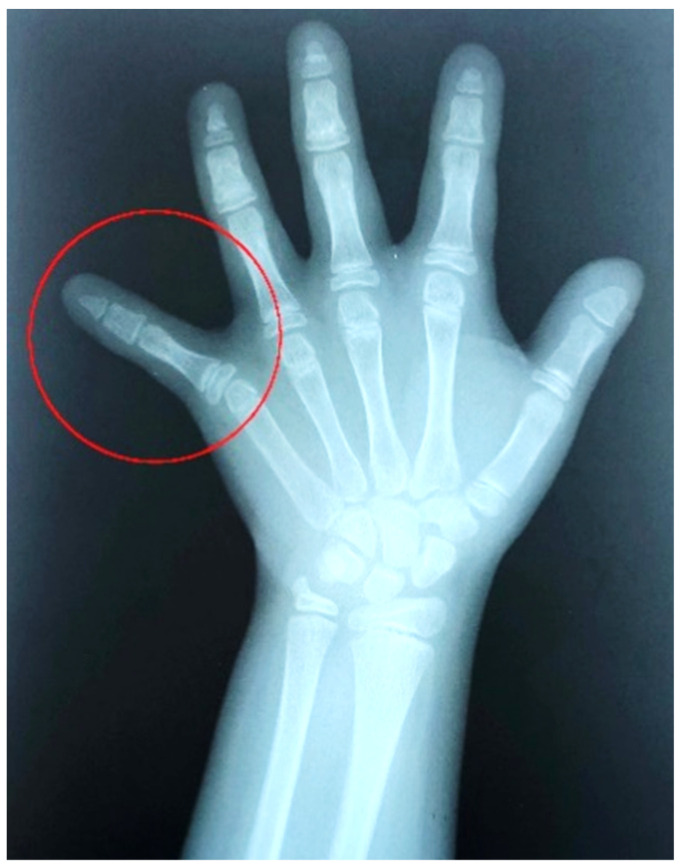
Initial 10 April 2008 left hand X-ray (10-year-old): joint alteration of the fifth finger of the left hand (red circle).
